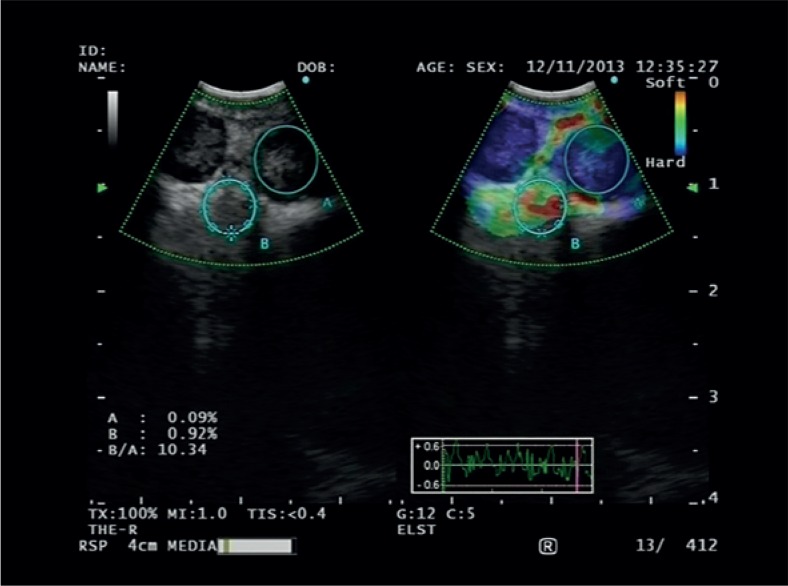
Endobronchial ultrasound (EBUS) elastography image of subcarinal lymph nodes. The image on the left-hand side displays greyscale ultrasound features. The image on the right-hand side is a superimposed elastographic image with a colour-coded scale (the hardest tissues are shown in blue and the softest in red). Symbol B/A represents the strain ratio, calculated between selected areas of the lymph node and the surrounding tissue.
